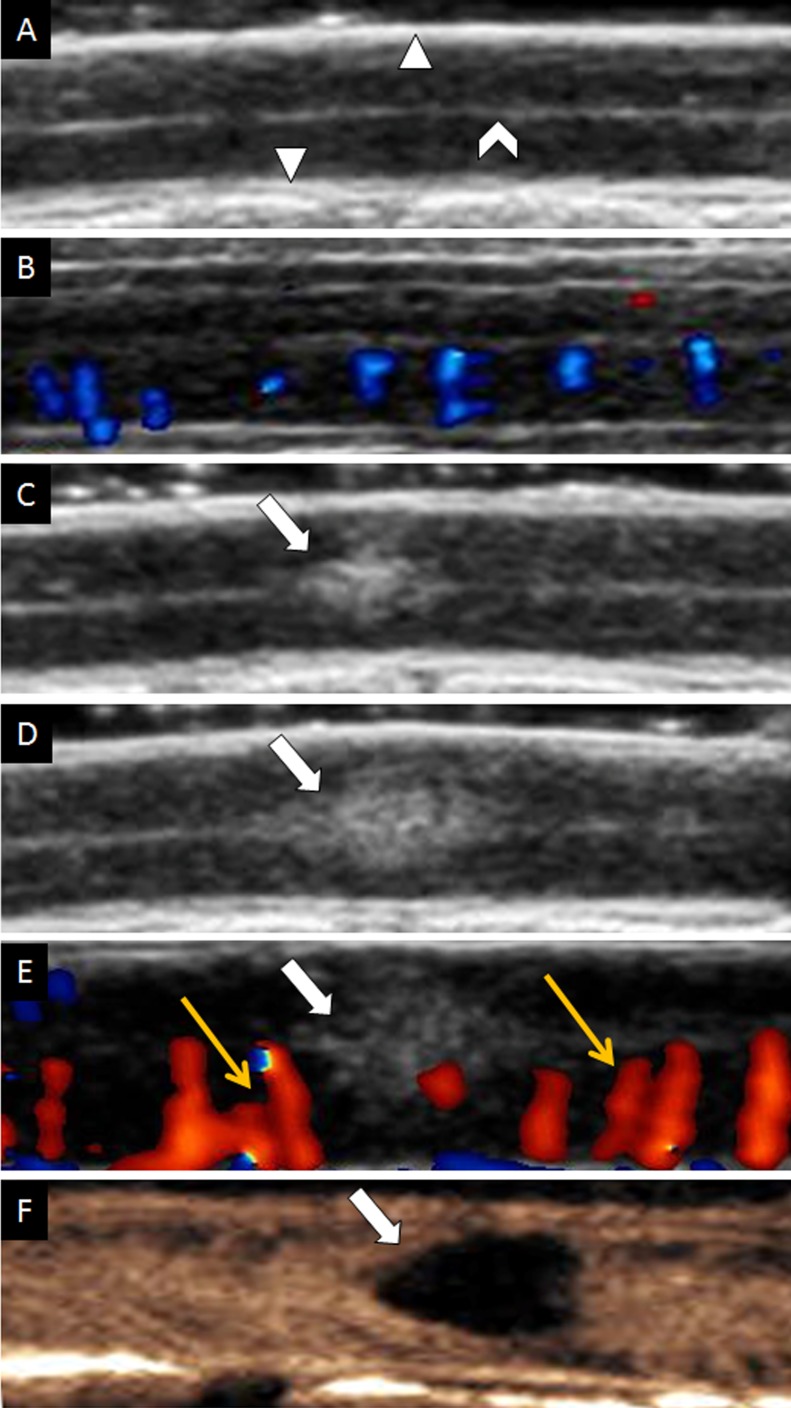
Ultrasound imaging of spinal cord on longitudinal view (A) Gray scale of uninjured spinal cord. (B) CDFI of uninjured spinal cord. (C) Contusive spinal cord instantly on gray-scale sonography. (D) Increasing size of contusive spinal cord on gray-scale sonography. (E) CDFI of injured spinal cord. Yellow arrows indicated dilated vessels. (F) CEUS showed the contusive lesion was non-perfusion. The white triangle indicated dura mater of spinal cord; The white caret indicated cerebral aqueduct. The white arrow indicated contusive lesion.
